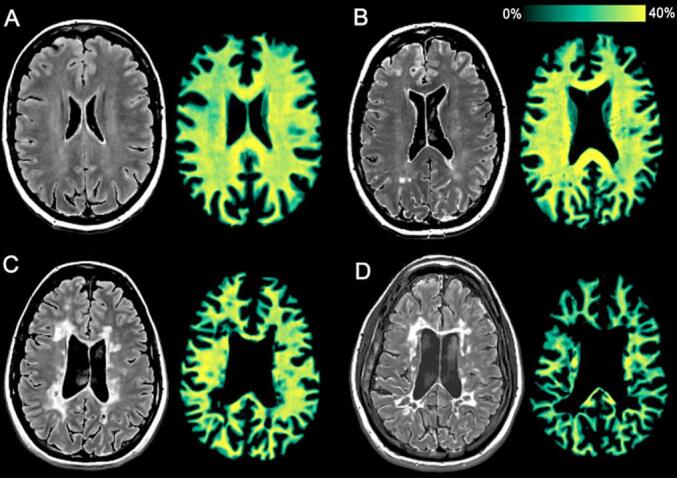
T2-weighted FLAIR and corresponding SyMRI myelin map in 4 subjects. A) A 56-year-old healthy control. B) A 53-year-old patient with primary progressive multiple sclerosis (MS). C) A 39-year-old patient with relapsing–remitting MS. D) A 40-year-old patient with secondary progressive MS. The myelin map shows the lowest values in the patient with secondary progressive MS, and the highest values in the healthy control. Adapted with permission from Ouellette et al., 2020.
